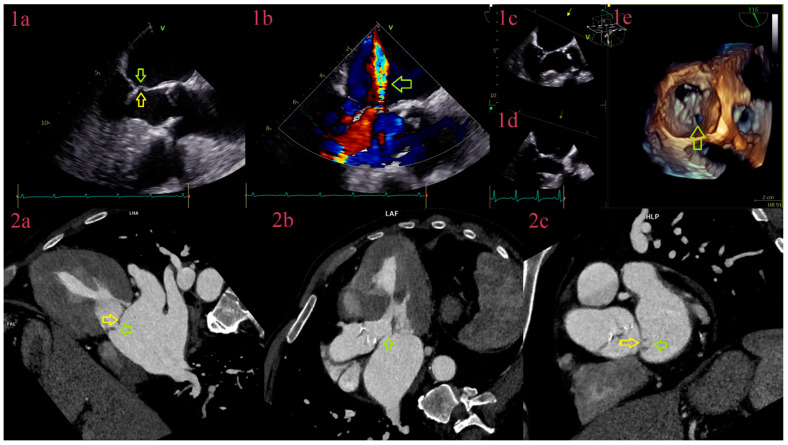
Perforation of anterior cusp of the mitral valve. Comparative overview of TEE and CCT in IE of the mitral valve. TEE, in 3-chamber view, depicts thickened aneurysm of anterior cusps (yellow arrow) with perforation (green arrow) (1a), confirmed with color Doppler flow with two-jet phenomenon (1b) and in volume-rendering reformation (1c–1e). CCT, in 3-chamber view, depicts aneurysm of anterior cusps (yellow arrow) with perforation at its top (green arrow) (2a,2b) and in the plane of the mitral valve (2c).
